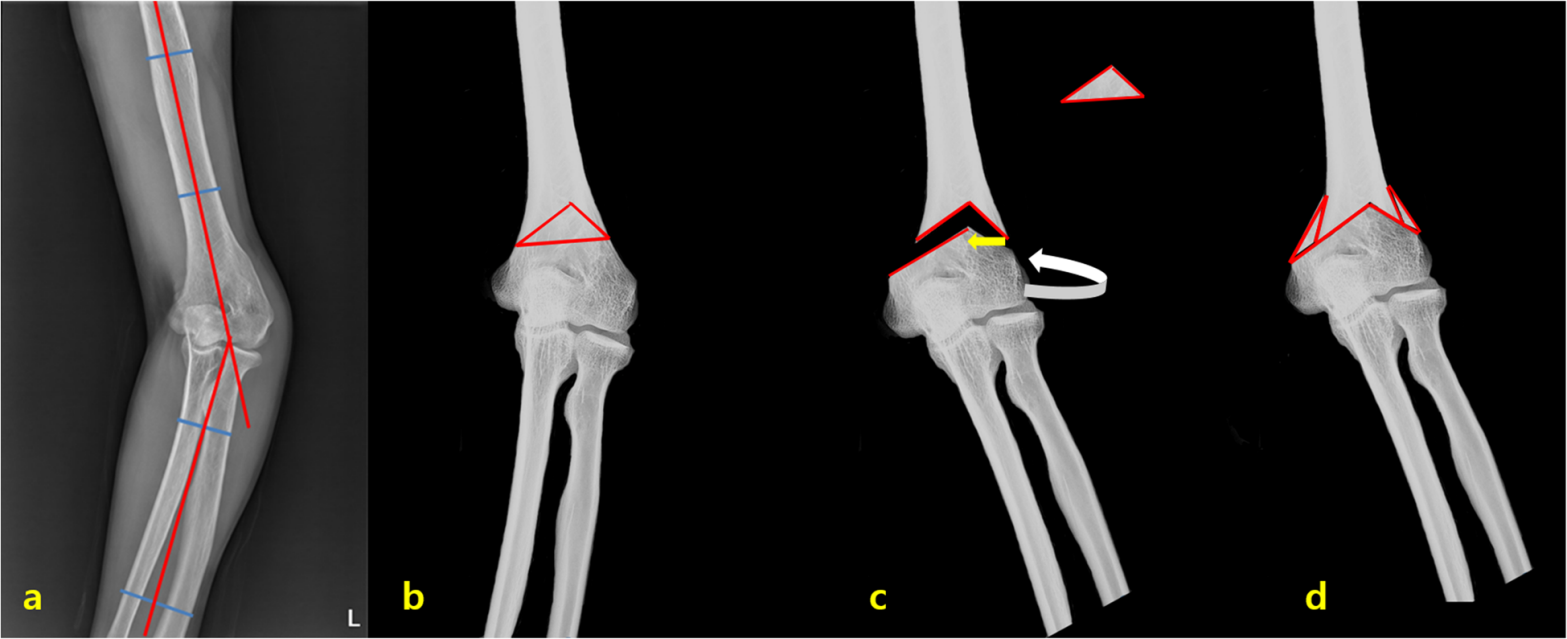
Translation step-cut osteotomy for correction of cubitus varus deformity. a. The humerus-elbow-wrist (HEW) angle is measured on an anteroposterior radiograph. b. After the correction angle is determined by comparing HEW angles of both elbows, the initial transverse osteotomy line is made about 0.5 to 1 cm superior to the olecranon fossa and perpendicular to the axis of the humerus. The osteotomy is performed along the triangular line. c. The deformity is corrected by rotating the distal fragment externally to correction internal rotational (white arrow) and translating it medially to prevent lazy S-shaped deformity (yellow arrow). d. After correction, the excised triangular bone is used as supplementary bone graft
